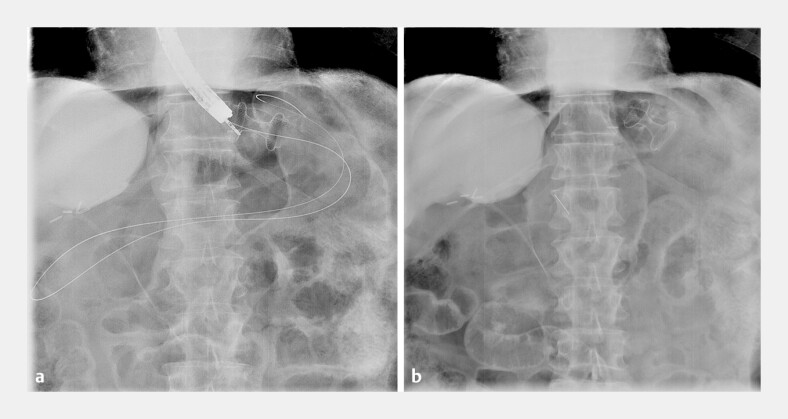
Radiographic images of the remove-and-replace technique being performed over a guidewire showing:
